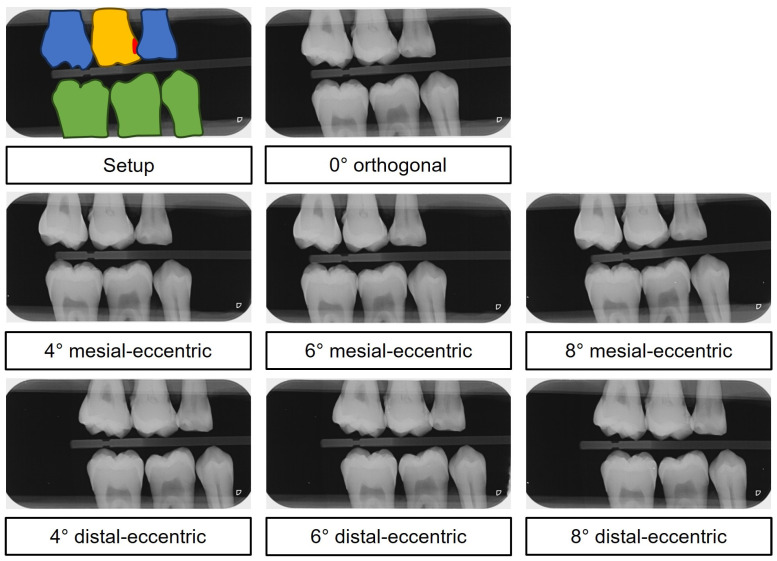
Digital in vitro bitewing images. Top: color-coded setup—yellow: examination tooth, red: carious lesion, blue: adjacent tooth, green: antagonistic tooth. Below: The mesial-eccentric series shows increased superimposition as the ray path becomes increasingly eccentric in the proximal region of teeth 46 and 47. Conversely, the distal-eccentric series shows increased superimposition as the ray path becomes increasingly eccentric in the interproximal region of teeth 15 and 16.
